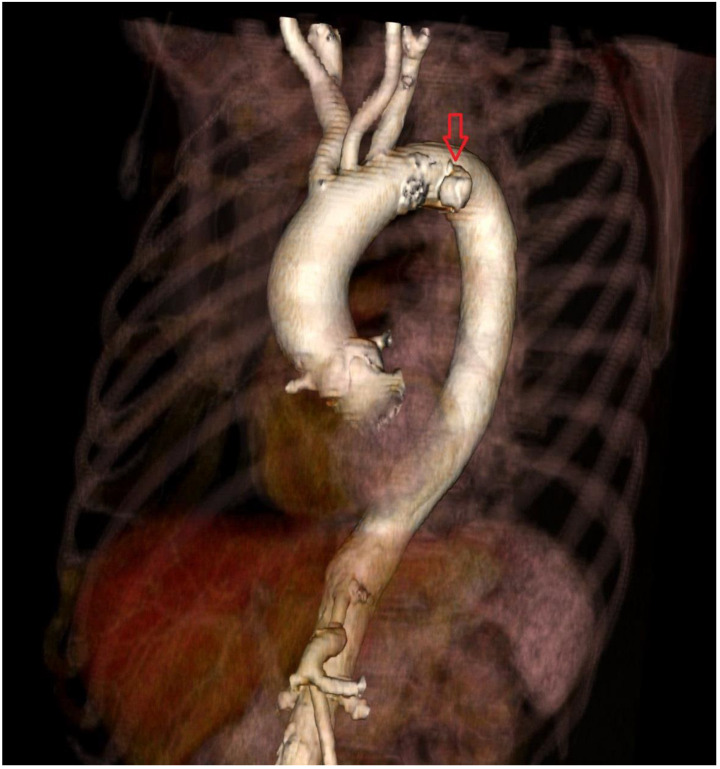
Preprocedural 3D CT; red arrow shows PAU.
